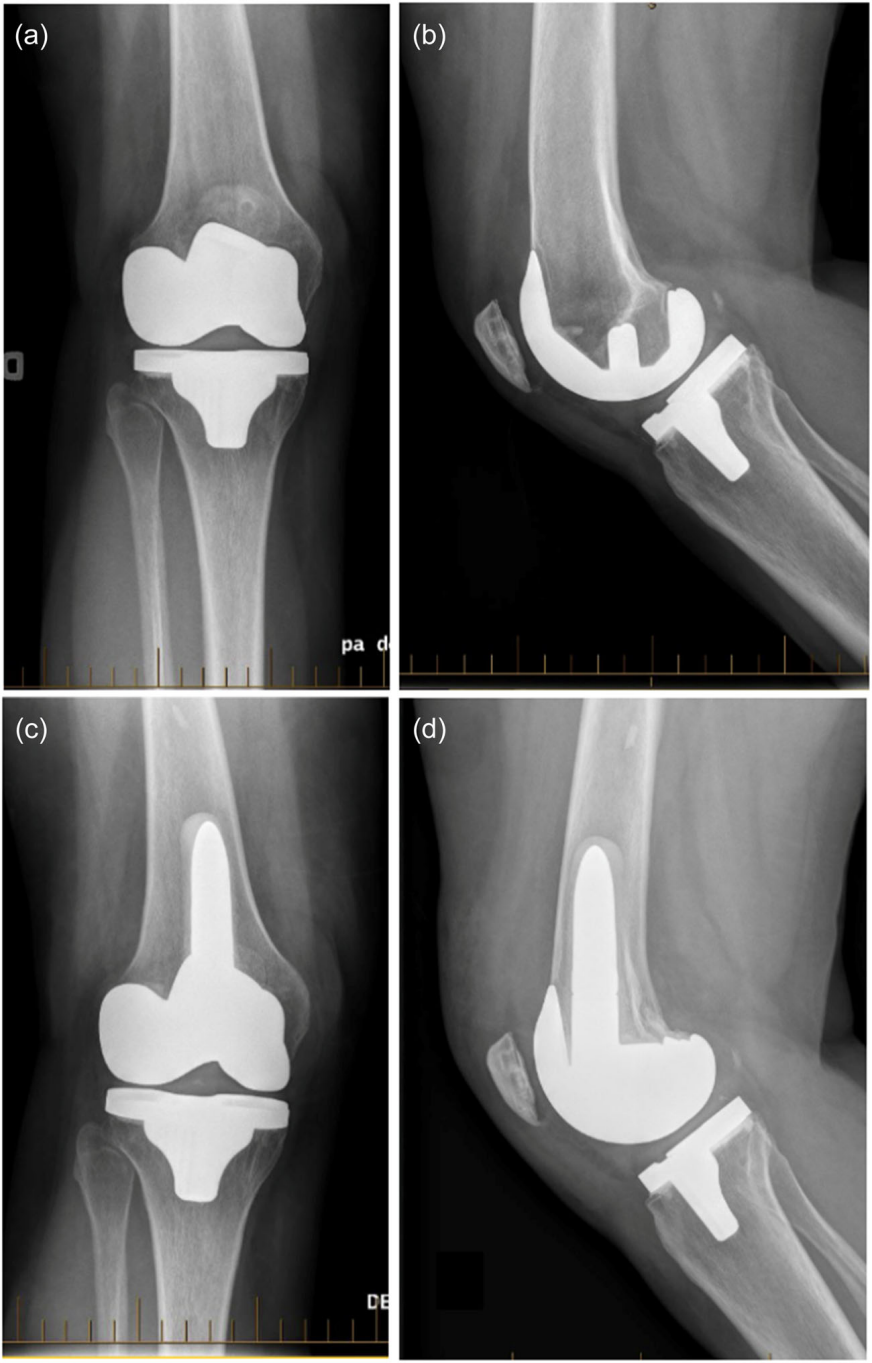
TKA instability and recurrent hemarthroses secondary to femoral component malrotation. Anteroposterior (a) and lateral (b) radiographs of the TKA before implant revision (femur 3° valgus, tibia 1° varus, HKA 2° valgus). Anteroposterior (c) and lateral (d) radiographs after revision TKA with posteromedial femoral augment and stem (femur 4° valgus, tibia 1° varus, HKA 3° valgus). Her knee phenotype did not change over the treatment process: VAL_FMA_3°/VAL_TMA_3°. HKA, hip knee ankle; TKA, total knee arthroplasty.
